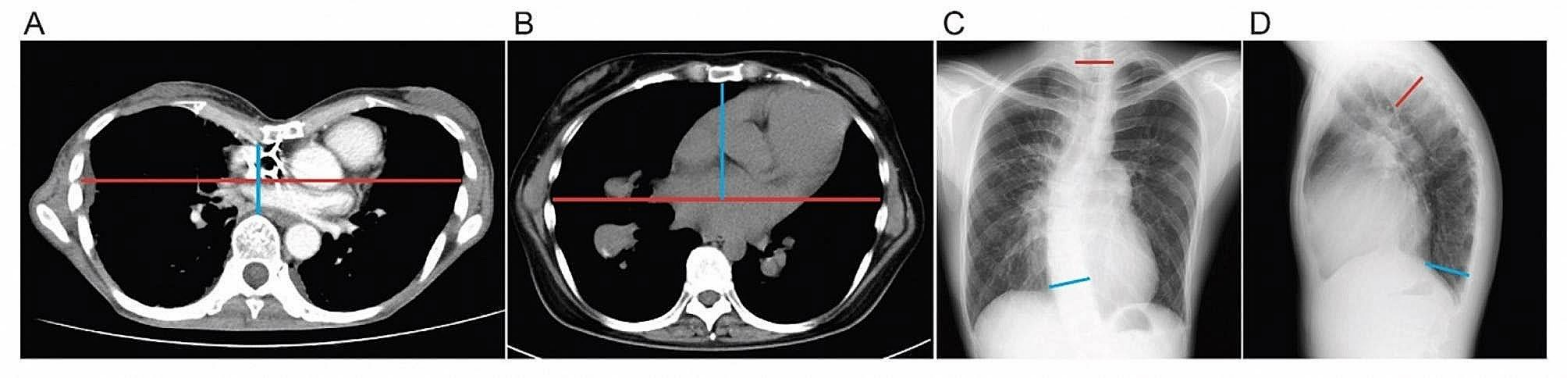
Measurement of thoracic and vertebral deformities and representative images. (A) Pectus excavatum was assessed using an axial chest CT image, and the Haller index was calculated by dividing the widest transverse diameter (red line) by the highest antero-posterior distance (blue line). (B) Pectus carinatum was assessed using an axial chest CT image, and the modified pectus index was calculated by dividing the widest transverse diameter (red line) by the distance from the central chord to the underside of the maximal protrusion (blue line). (C) Scoliosis was assessed using a posteroanterior chest radiograph, and the Cobb angle was calculated between the second and 11th thoracic vertebrae (shown by the red and blue lines, respectively). (D) Kyphosis was assessed using a lateral chest radiograph, and the Cobb angle was calculated between the fourth and 12th thoracic vertebrae (shown by the red and blue lines, respectively)
